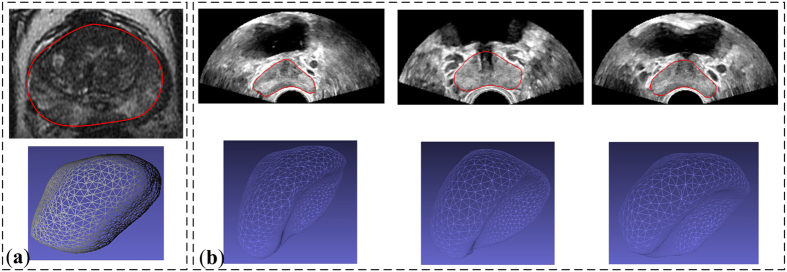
MR and TRUS prostate images. (a) MR image and 3D prostate surface model without probe-induced deformation, (b) TRUS images and 3D surface models with various probe-induced deformations.
